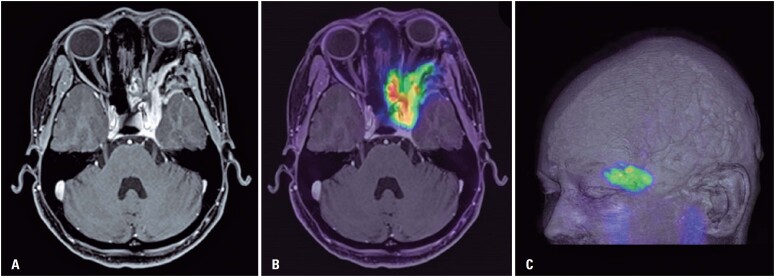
Example of a skull base meningioma with high somatostatin analog uptake. The tumor extends to the cavernous sinus and the left orbital and nasal cavities. Axial magnetic resonance image contrast enhanced T1-weighted sequence (A); PET/MRI fusion image (B) and 3D volume rendering (C)
